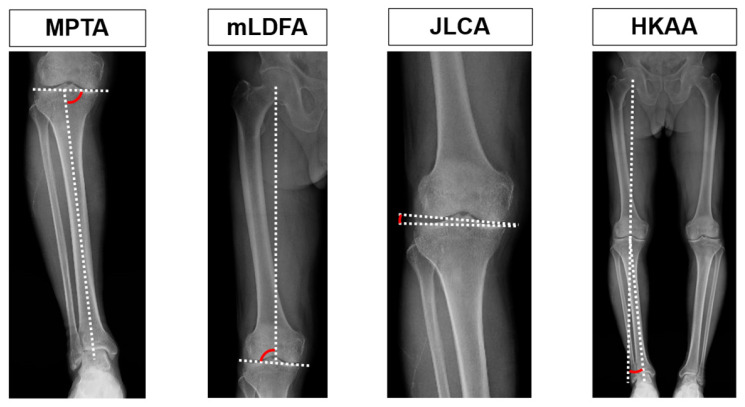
Radiographic definitions of coronal alignment parameters.
